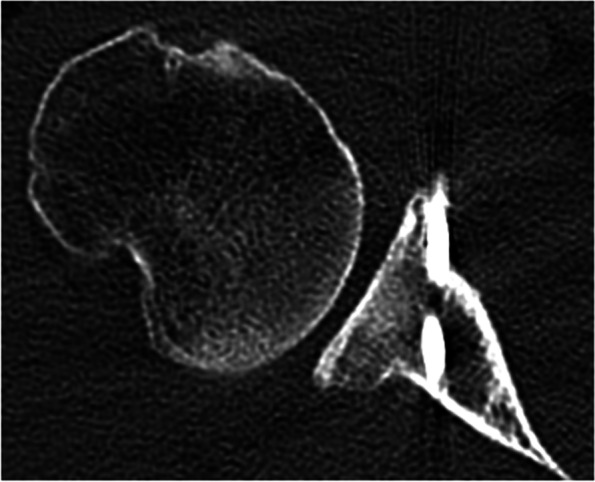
Postoperative CT-scan of a patient with a large and medial Hill-Sachs lesion that was not fully restored with the Latarjet procedure
